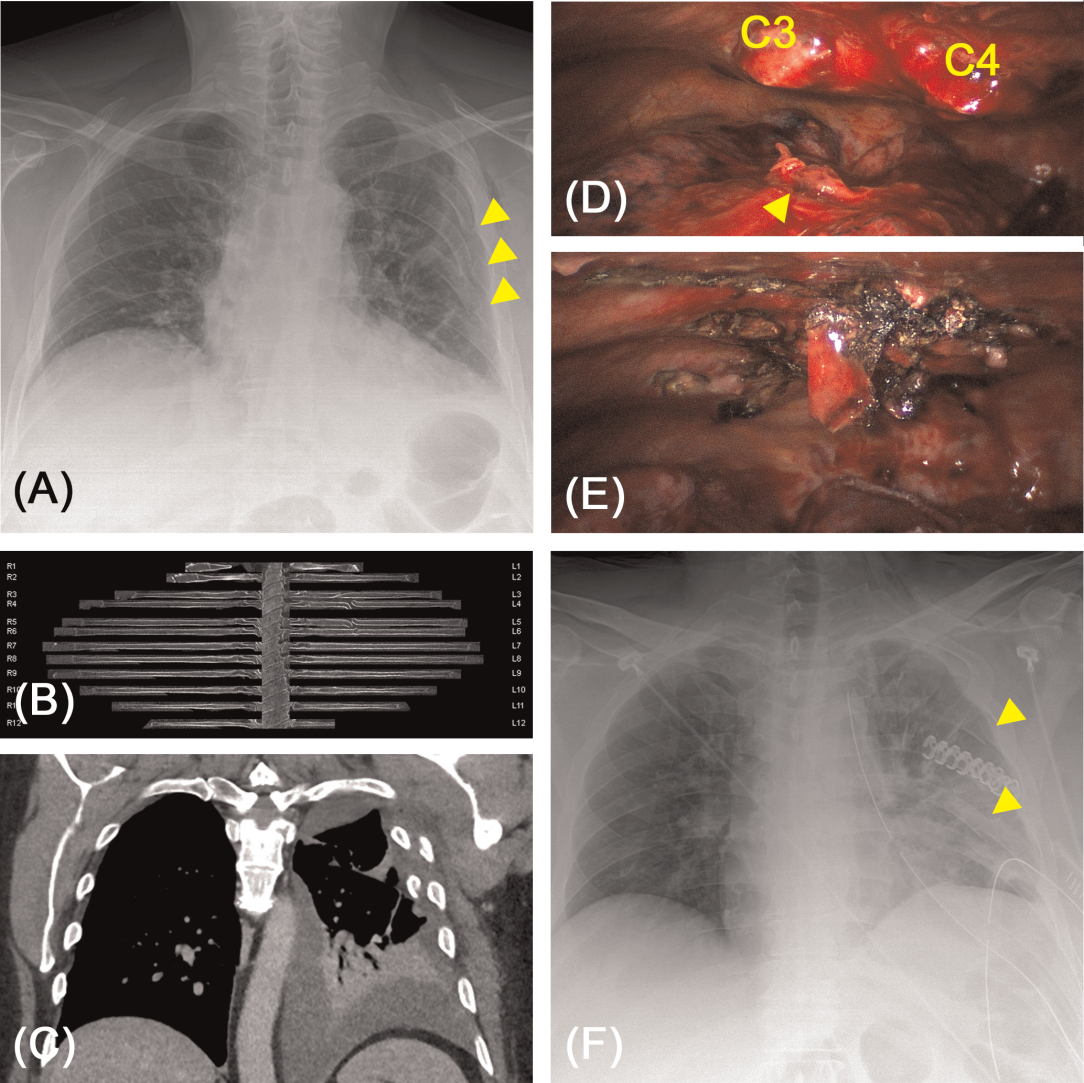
(A) 59-year-old-patient after fall. Sudden hemodynamic instability six days after the accident. Chest X-ray revealed secondary dislocation of rib fractures (arrows). (B,C) Computed tomography including curvilinear reformation confirmed the dislocation of fracture endings causing a novel hemothorax. (D,E) Intraoperative videoscopy showed dislocated fracture endings of costae 3 and 4 subscapular causing an actively bleeding laceration of the lung parenchyma (arrow). After osteosynthesis of costa 4 the lateral chest wall was sufficiently erected and palpatory stable. (F) Post-operative chest X-ray after rib stabilization, reconstruction of lung parenchyma and relieve of 800 mL hemothorax. By a single stabilization the adjacent ribs were also repositioned (arrows).
